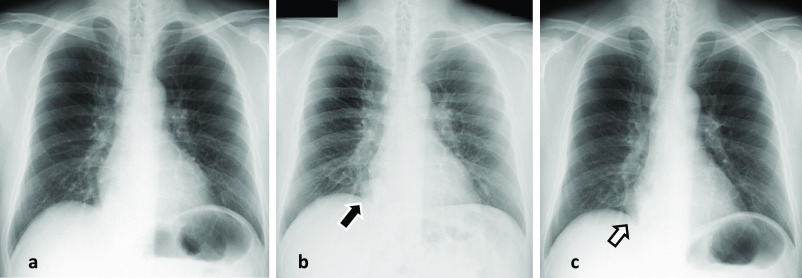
Chest X-ray a. taken 2 years before presentation is normal. b. taken 1 year before presentation shows a coin lesion in the right lower lung
